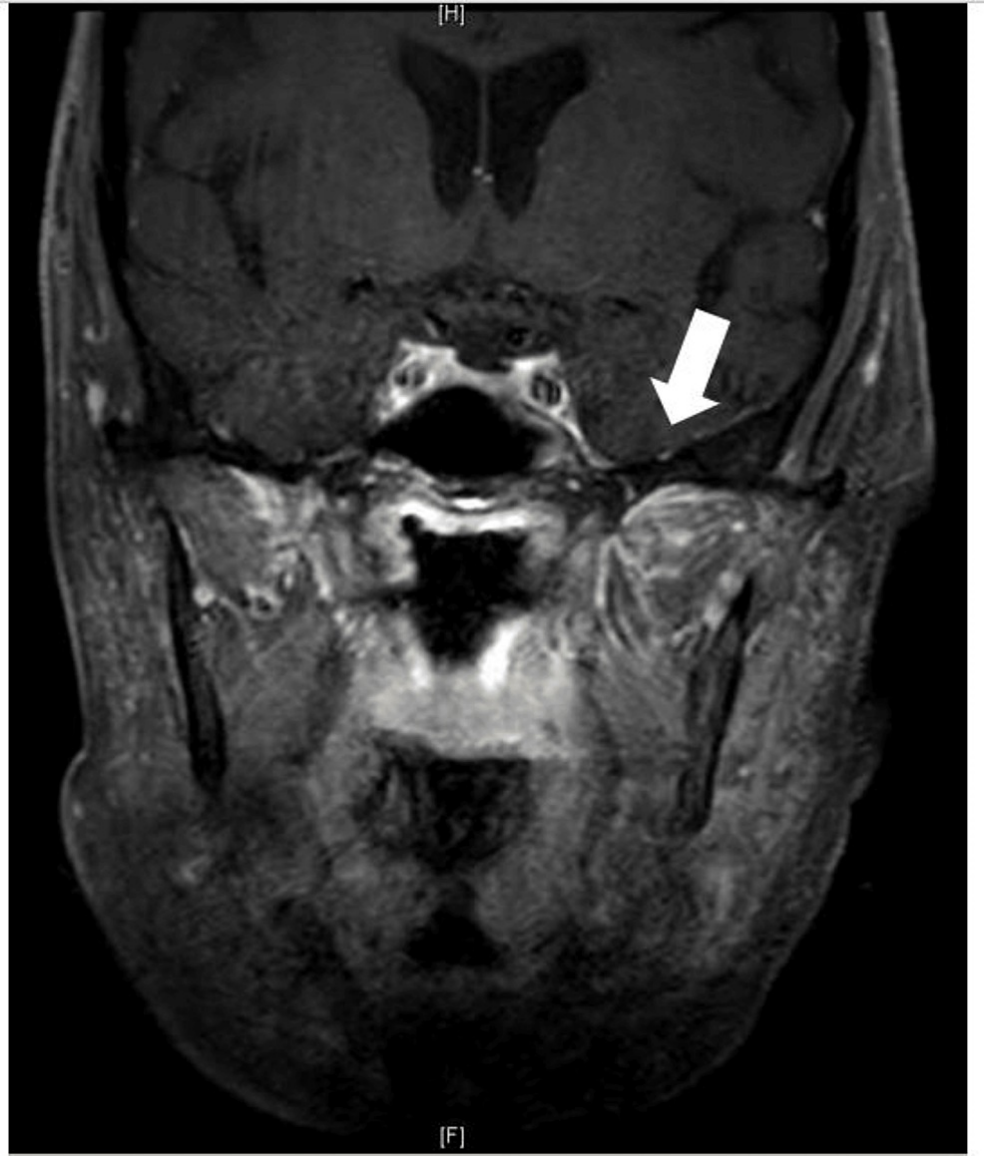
Patient’s last MRI which revealed a persistent abnormal signal and enhancement in the left pterygomaxillary fissure and left foramen rotundum although mild dural enhancement along the left medial middle cranial fossa had decreased (arrow).
